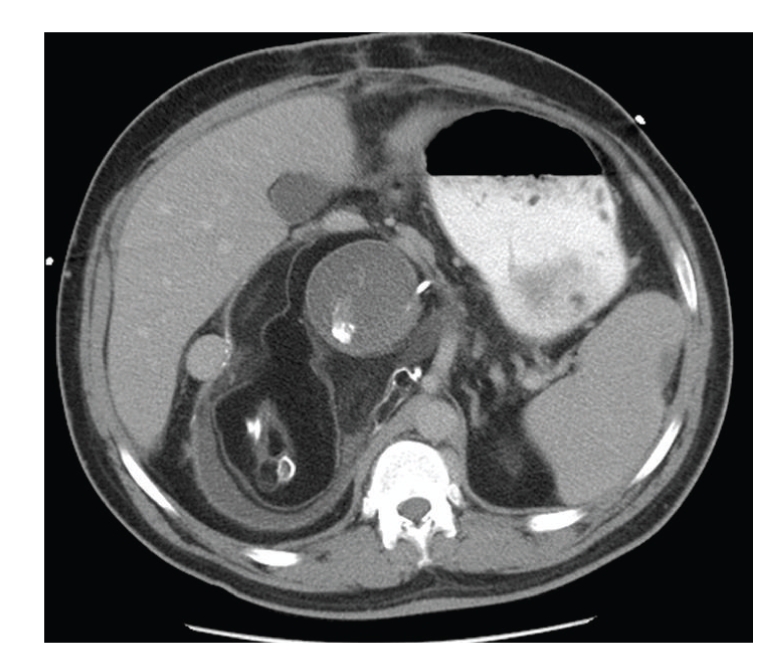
CT scan with contrast shows a retroperitoneal mass containing bone, soft tissue, and cystic elements.
